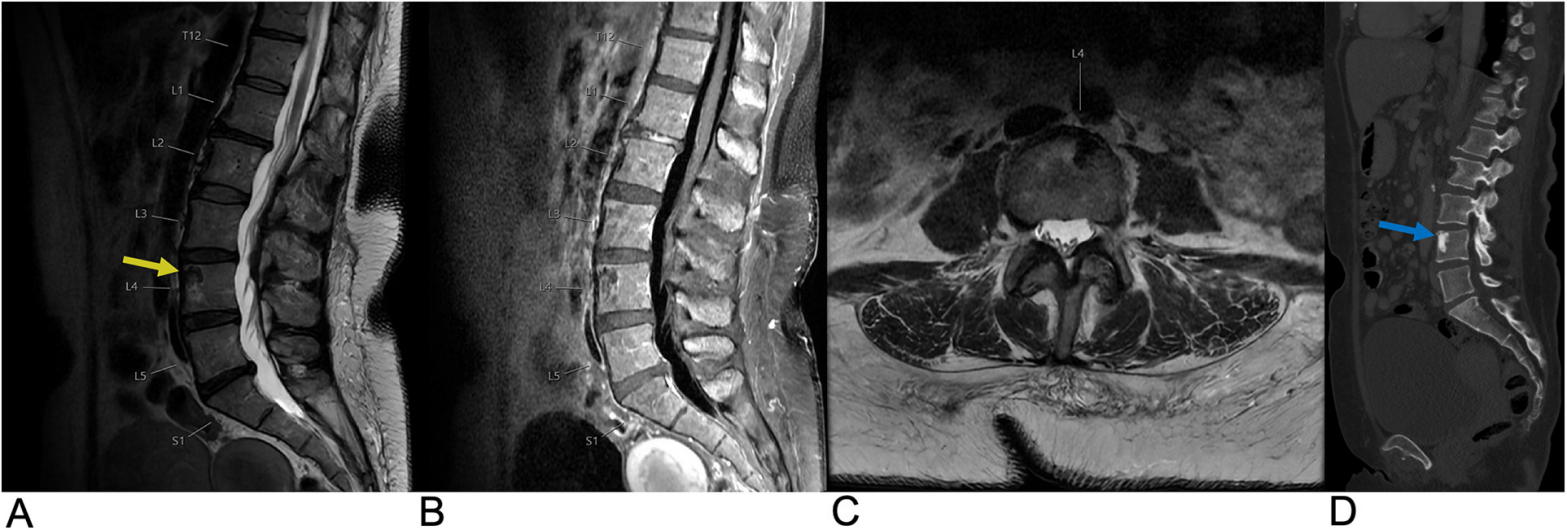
Enostosis. Sagittal T2 (A), sagittal T1 FLAIR FAT + C (B), and axial T2 (C) MRI as well as a sagittal CT (D) of the lumbar spine demonstrating a T1/T2 hypointense, sclerotic (yellow arrow) and CT hyperdense (blue arrow) lesion within the left anterior aspect of the L4 vertebral body representing enostosis.
